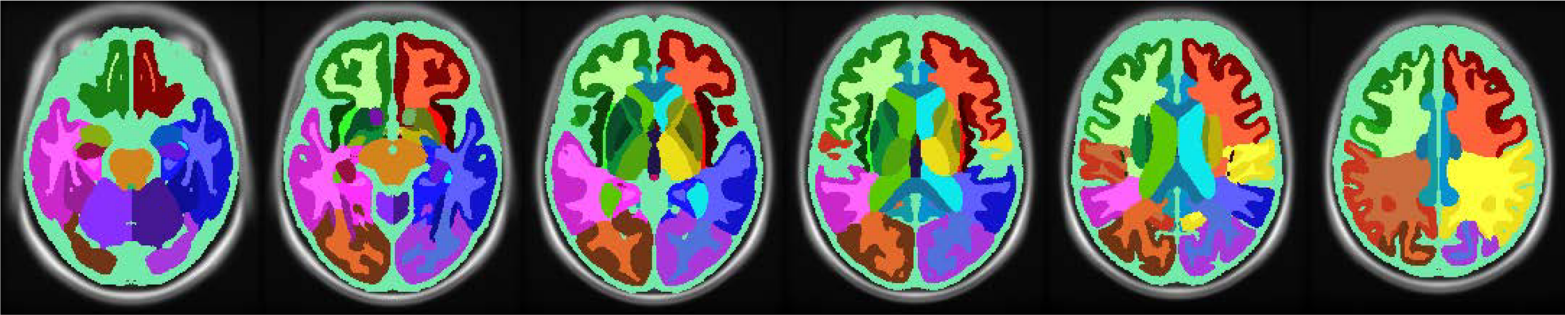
Anatomical atlas for regional segmentation. Regional segmentation was done by non-linearly warping an atlas of 56 regions to the T1-weighted image.
